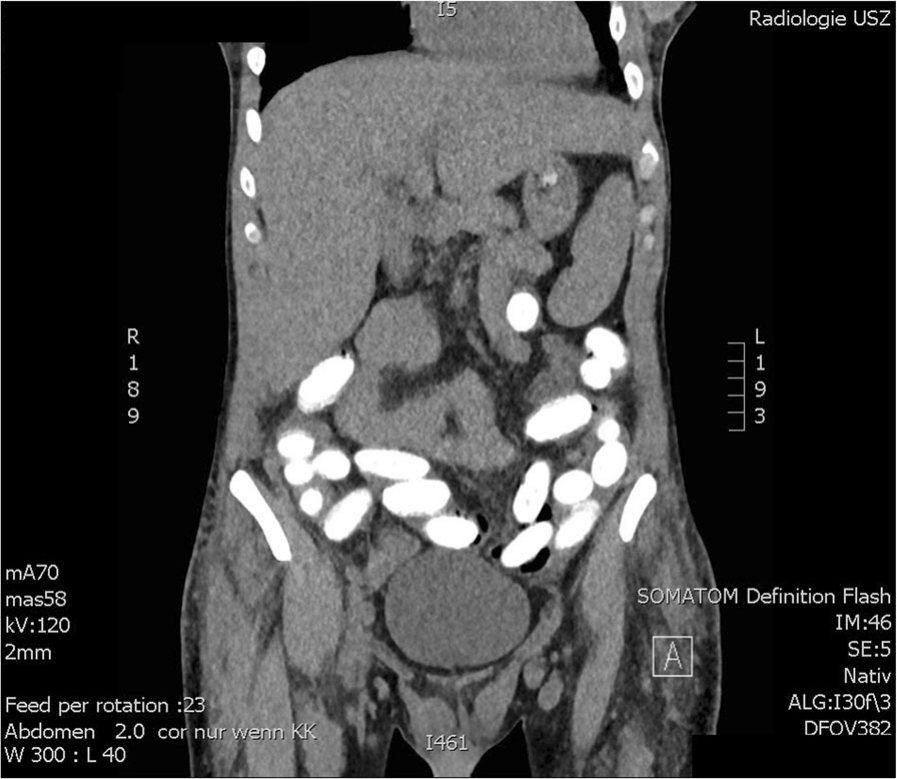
Computed tomography scans at the initial presentation showing multiple drug packages in the patient’s gastrointestinal tract
